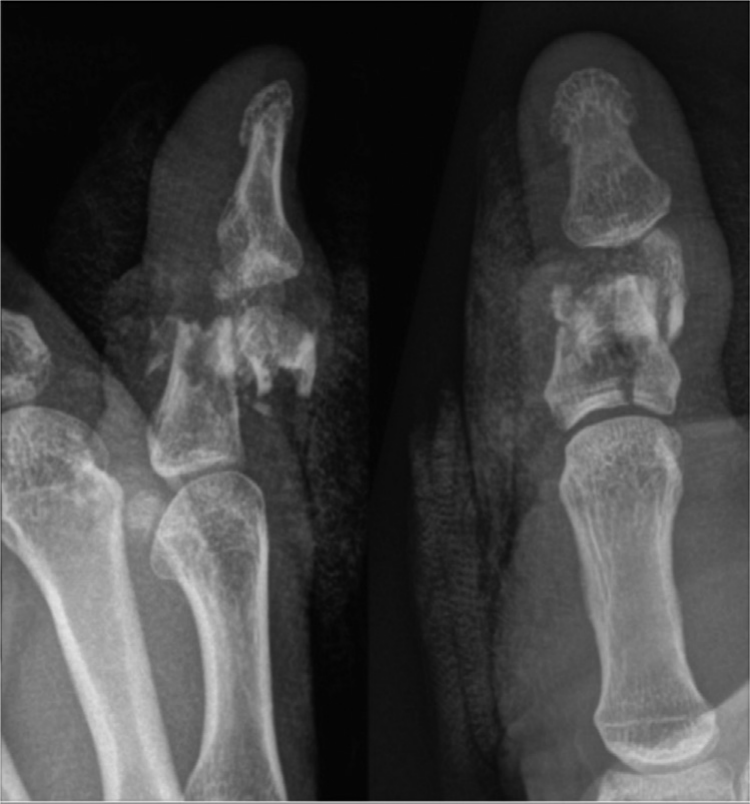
Plain film x-ray demonstrated a comminuted intraarticular fracture of the head and base of the proximal phalanx.
